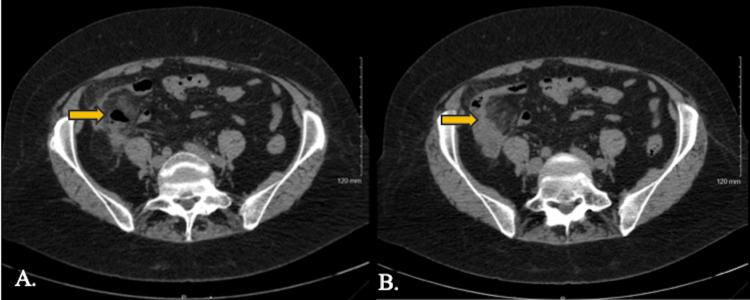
Axial CT scan of the abdomen and pelvis five days after the patient’s presentation in the office. Image A is cephalad to image B. The arrow in A depicts diverticular outpouching in the distal ileum. The arrow in B indicates amorphous fat stranding surrounding the distal ileal loop.
